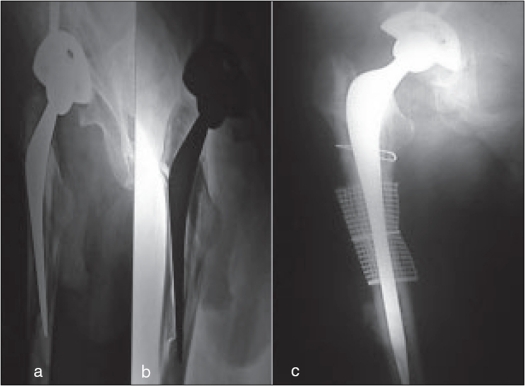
(a-c) X-rays of a patient with periprosthetic fracture six years after index surgery due to roadside accident. Femoral component revised with long stem
